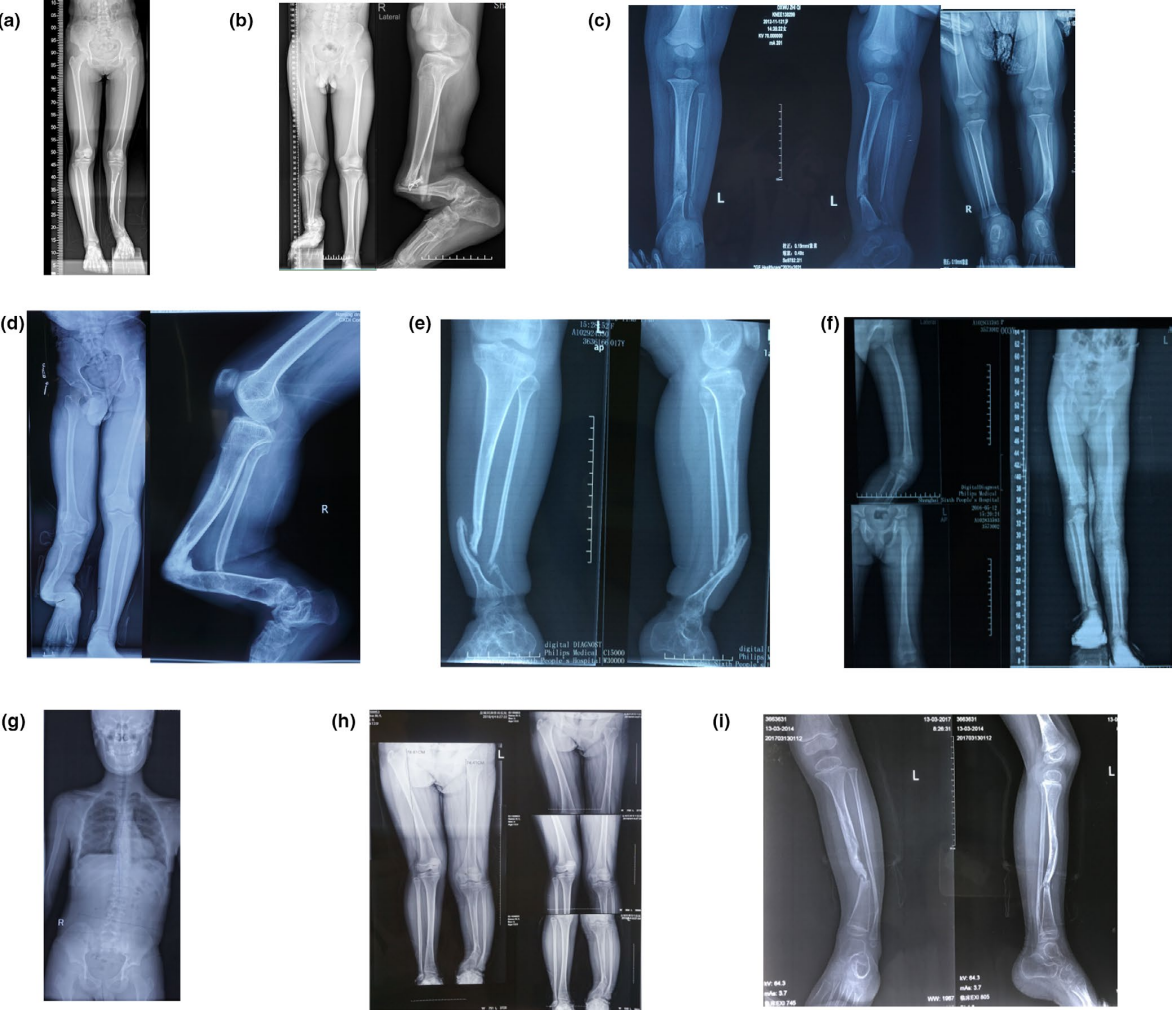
The radiological evidence of NF1 in our patients. (a) II‐1 of family 1: the radiograph demonstrates her congenital pseudarthrosis of the left tibia. (b) II‐2 of family 2: the radiographs demonstrate his congenital pseudarthrosis of the right tibia. (c) III‐2 of family 3: the radiographs demonstrate her congenital pseudarthrosis of the left tibia. (d) III‐6 of family 4: the radiographs demonstrate his congenital pseudarthrosis of the right tibia. (e) III‐3 of family 5: the radiographs demonstrate her congenital pseudarthrosis of the left tibia. (f) III‐2 of family 7: the radiographs demonstrate his unequal leg length deformity. (g) II‐2 of family 8: the radiograph demonstrates his scoliosis. (h) III‐2 of family 9: the radiograph demonstrates her tibial bowing deformity. (i) IV‐1 of family 3: the radiographs demonstrate her congenital pseudarthrosis of the left tibia. All of the images are published with permission from the affected individuals
